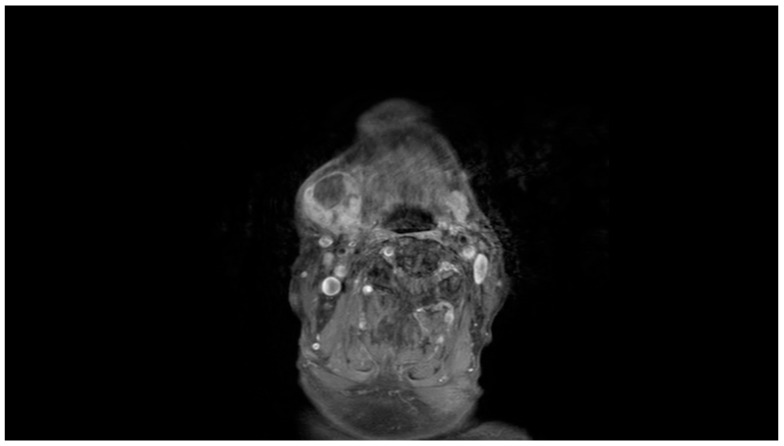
Coronal magnetic resonance image of the neck demonstrating a heterogeneous mass in the right submandibular region, involving the submandibular salivary gland and adjacent soft tissues.
